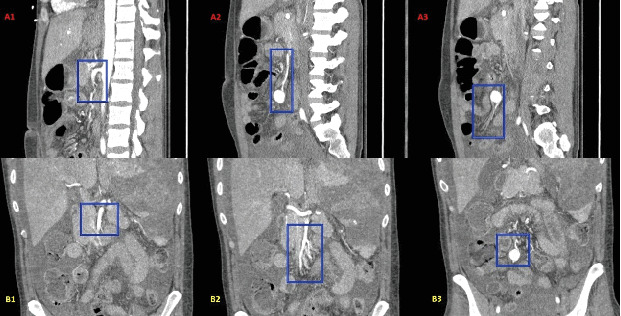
CTA of the abdomen and pelvis showing the course of the superior mesenteric artery and pseudoaneurysm in the sagittal and coronal views. (a1) Sagittal view of the abdomen with a blue rectangle outlining the origin of the superior mesenteric artery and adjacent abdominal aorta. (a2) Sagittal view of the abdomen with a blue rectangle outlining the proximal superior mesenteric artery and pseudoaneurysm. (a3) Sagittal view of the abdomen with a blue rectangle outlining the superior mesenteric artery pseudoaneurysm and distal arterial branches. (b1) Coronal view of the abdomen with a blue rectangle outlining the origin of the superior mesenteric artery and adjacent abdominal aorta. (b2) Coronal view of the abdomen with a blue rectangle outlining the proximal superior mesenteric artery and pseudoaneurysm. (b3) Coronal view of the abdomen with a blue rectangle outlining the superior mesenteric artery pseudoaneurysm and distal arterial branches.
